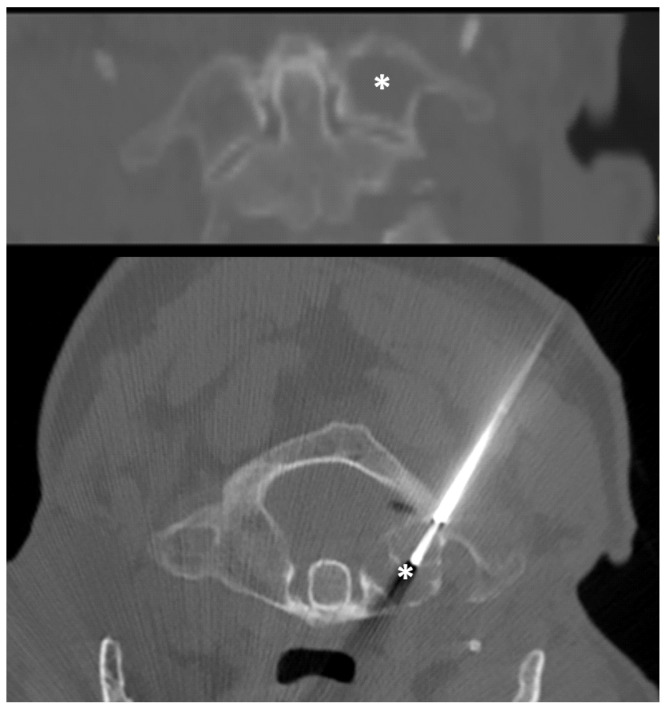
A 73-year-old man. CT frontal view (Up) showed a mixed lesion (mostly lytic, shown in asterisks) of the left lateral mass of the atlas. CT-guided biopsy was performed with a posterior approach (Down). The procedure resulted in the diagnosis of metastasis of prostate cancer.
